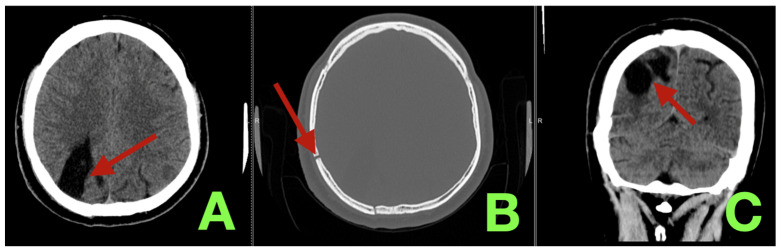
Immediate postoperative non-contrast CT. (A): Axial parenchymal window showing a sharply delimited right parieto-occipital postoperative cavity (arrow) replacing the evacuated hematoma and excised AVM nidus, containing expected low-density CSF/pneumocephalus admixture with a thin rim of reactive peri-cavitary hypodensity and no hyperdense re-bleed or new mass effect. (B): Axial bone window confirming anatomically stable right parieto-occipital craniotomy margins (arrow) with a re-seated flap and no early epidural or subdural collection. (C): Coronal reconstruction demonstrating the posterior convexity resection bed (arrow) with a small air–fluid level typical of immediate postoperative pneumocephalus, preserved hemispheric symmetry, and no evidence of acute hydrocephalus or renewed midline shift.
